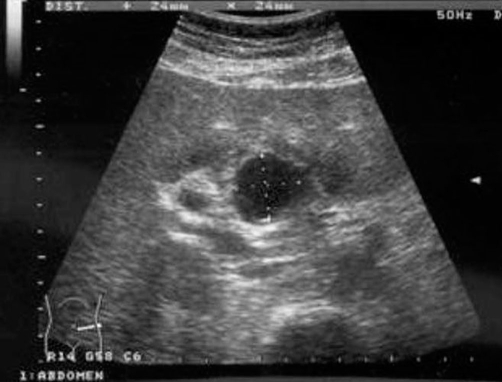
Ultrasound scan showing aneurysmal sac to the left of the common bile duct and portal vein.
